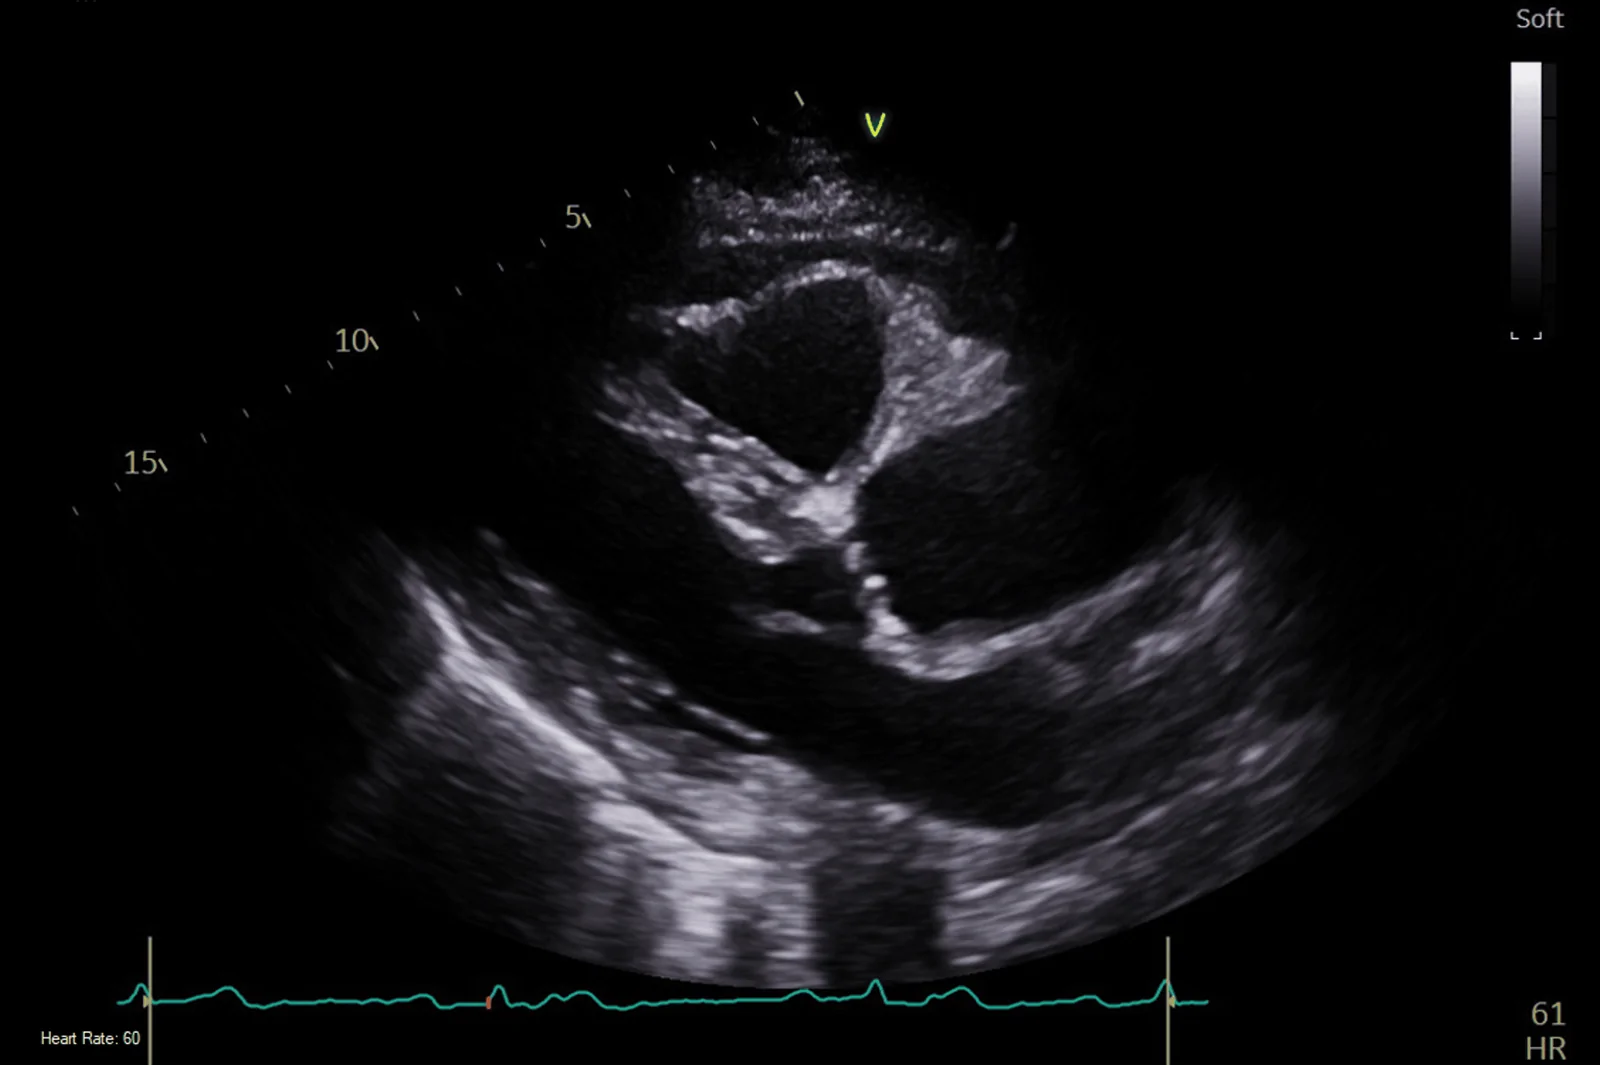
Transthoracic echocardiography (TTE) image showing pericardial effusion.
